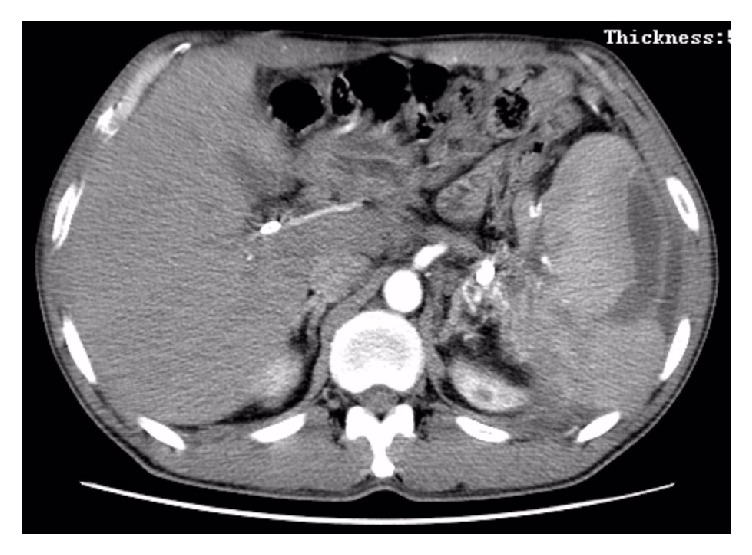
CT scan showing the volume of splenic subcapsular hematoma significantly reduced compared to all previous images.
